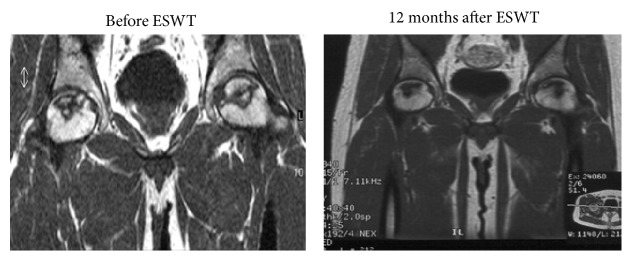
Radiographs of the bilateral hips before and after treatment in a 21-year-old male patient with osteonecrosis of the femoral head showing a trend of decrease in the size of the lesion after ESWT and no changes in the stages of the disease and no further collapse of the femoral heads.
